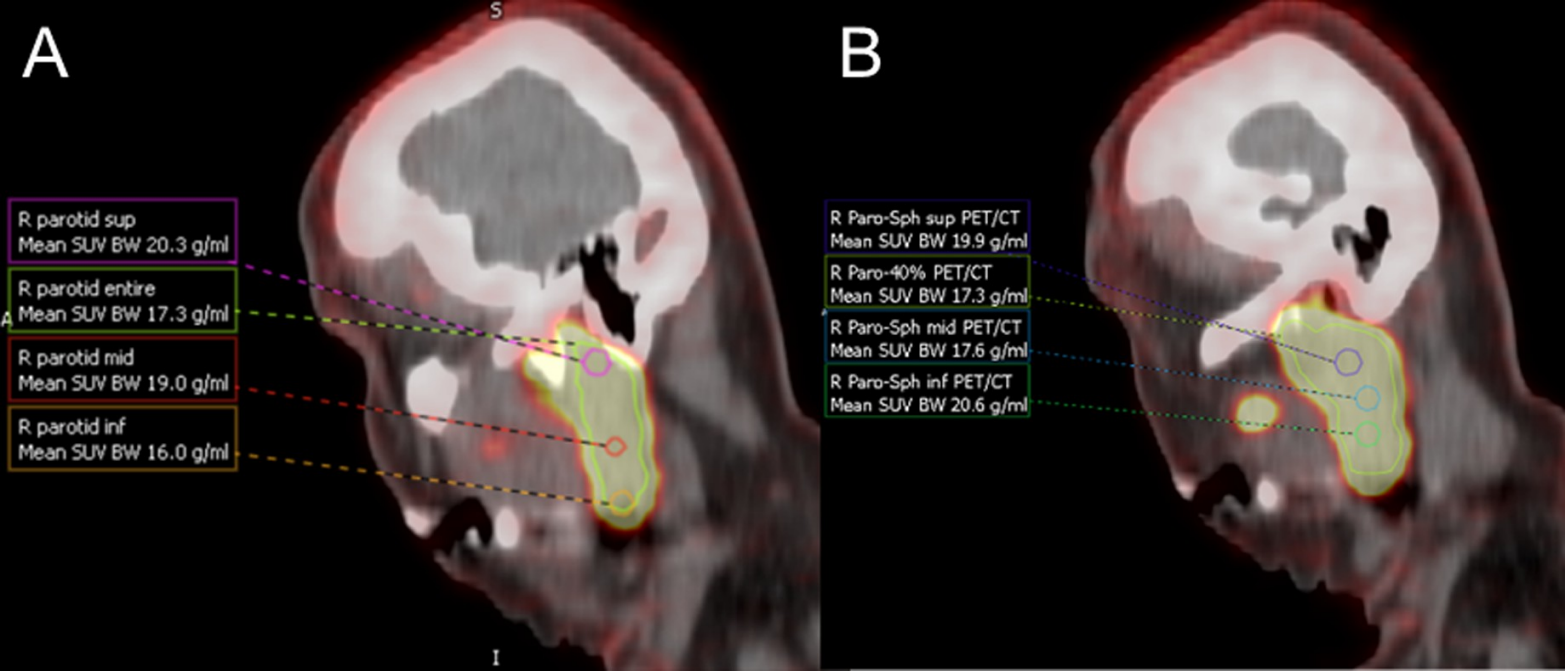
Example demonstrating variability for parotid region of interests (ROIs). Sagittal maximum intensity projection from the PET/MR [^18^F]DCFPyL PSMA tracer acquisition with labeled parotid gland and liver ROIs. Spatial variability of calculated SUV_mean_ for the 1-cm ROIs obtained from the superior (sup), middle (mid), and inferior (inf) right parotid gland is seen with values of 18.6, 22.4, and 21.0 g/mL respectively.
